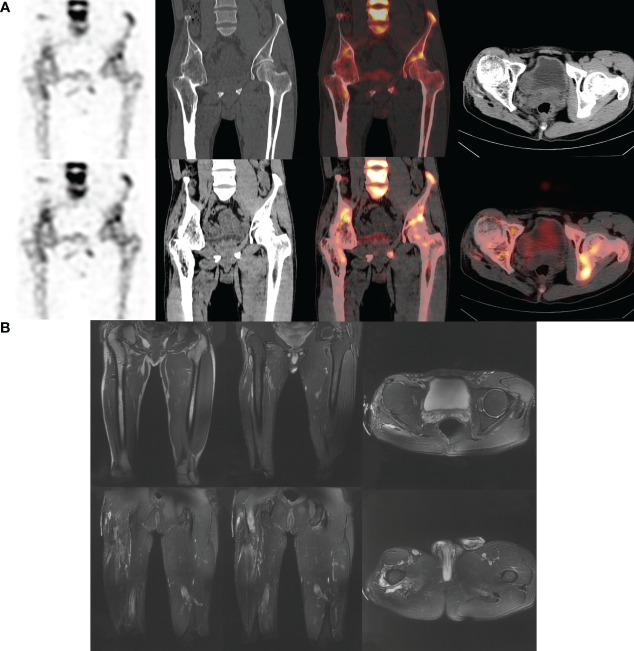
Male. 43y. There is no obvious cause of right lower limb pain, local swelling with pus discharge, and the pain worsens after activity. No fever. More than 10 years ago, the right femoral shaft fracture underwent reduction and internal fixation surgery and was removed. The physical examination was negative. WBC: 8.37×10^9^/L; LYM%: 16.7%; MONO%: 6.7%; NE%: 74.9%; ESR: 16.0 mm/hr; hs-CRP: 0.69 mg/L. Pathological examination showed infiltration of neutrophils, visible necrosis and abscess formation, and inflammatory changes. (A) SPECT/CT shows bone changes in the right proximal femur and right hip joint area with abnormal bone metabolism, which is consistent with chronic infectious disease. (B) MRI considers bony fusion of the right hip joint; Bone protrusions in the upper and middle segments of the right femur, considering ectopic ossification; Edematous changes in the right soft tissue.
